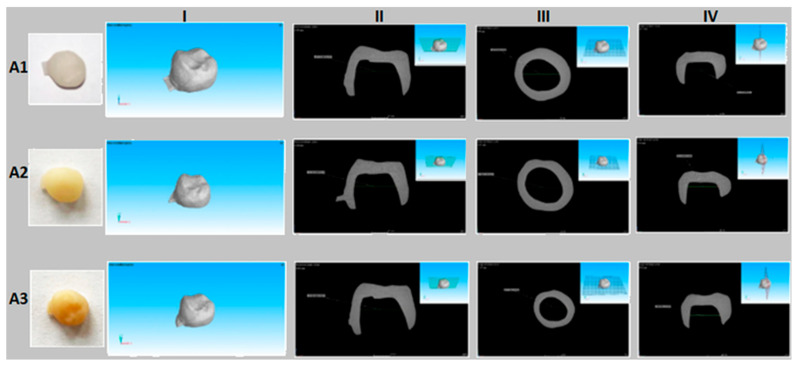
CT images of the analyzed samples. CT images of CAD/CAM-processed ceramic restorations: (A1,I–IV) zirconia-reinforced lithium silicate, (A2,I–IV) feldspathic ceramic, and (A3,I–IV) lithium disilicate.
